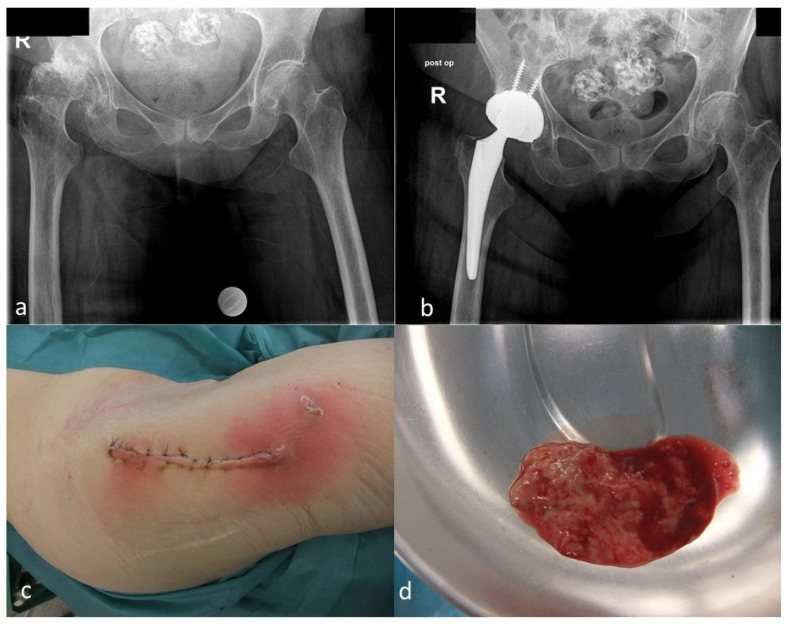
(a): Preoperative a.p. radiographs of the pelvis of a 79-year old female patient with a femoral head necrosis and secondary osteoarthritis of the right hip joint; (b): Postoperative radiographs after implantation of a cementless total hip arthroplasty; (c): Local findings 7 days after the surgery, indicating an early postoperative infection; (d): Purulent fluid was present in the joint (*Clostridum difficile*). The patient could be successfully treated by DAIR.
